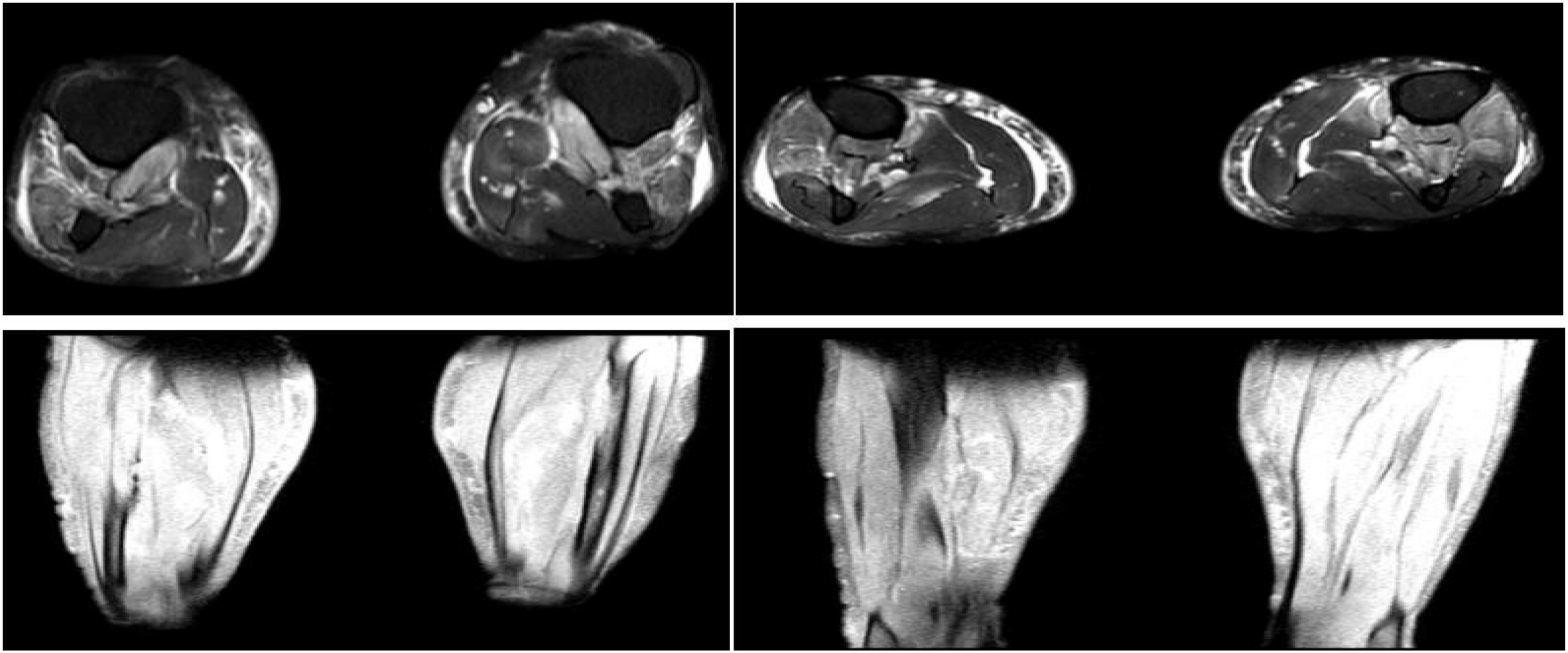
Bilateral lower leg muscles and subcutaneous soft tissues show extensive swelling with patchy slightly long T1 and slightly long T2 signal changes, accompanied by widespread increased and thickened vascular shadows. This non-contrast MRI of both calves strongly suggests active dermatomyositis, particularly the classic imaging triad of combined muscle plus subcutaneous tissue involvement coupled with vascular proliferation.
